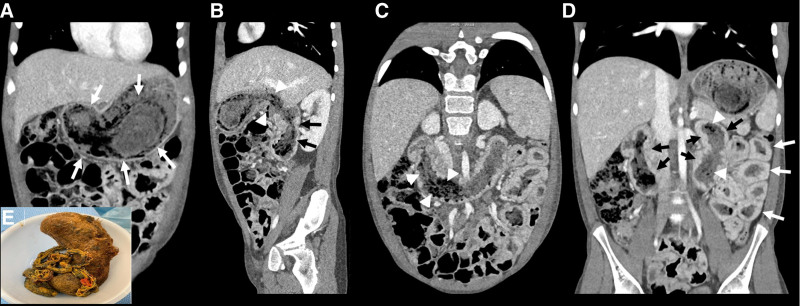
Coronal (A) and sagittal oblique (B) contrast-enhanced computerized tomography (CT) images of the abdomen and pelvis revealed a large, mixed attenuation mass distending the gastric lumen (white arrows) and extending through the pylorus (white arrowheads) into the proximal duodenum (black arrows). Coronal (C) and sagittal oblique (D) contrast-enhanced CT images of the abdomen and pelvis revealed a large, mixed attenuation mass distending the gastric lumen (white arrows) and extending through the pylorus (white arrowheads) into the proximal duodenum (black arrows). (E) Surgically extracted trichobezoar forming a cast of stomach, duodenum, and jejunum.
